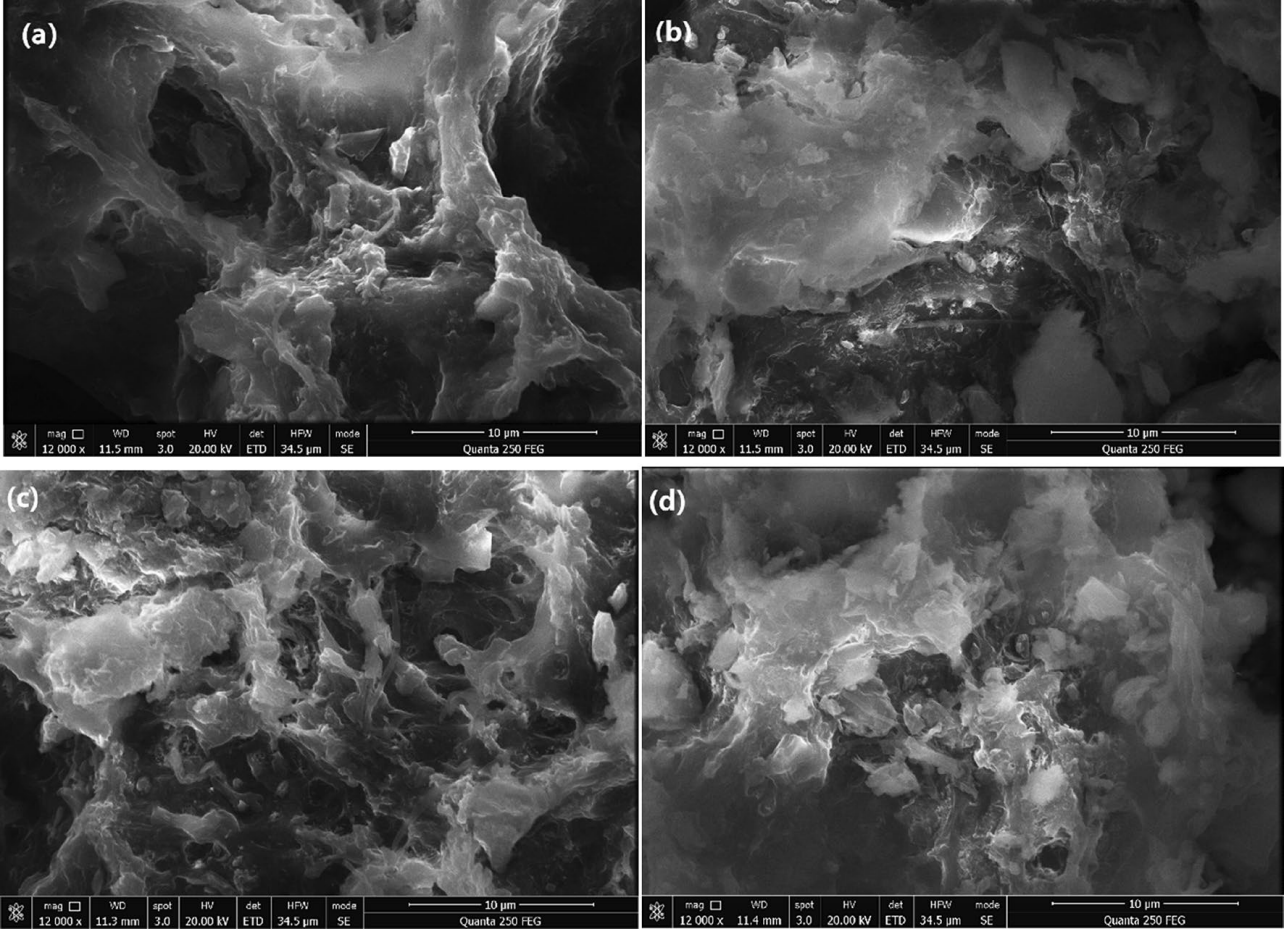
SEM images of sludge cakes: (a) raw sludge and conditioned by (b) ATP, (c) St-CTA, and (d) St-CTA in conjunction with ATP at respective optimal dose, that is, St-CTA is 16.00 mg/gTSS and ATP is 14.00 kg/m^3^.
